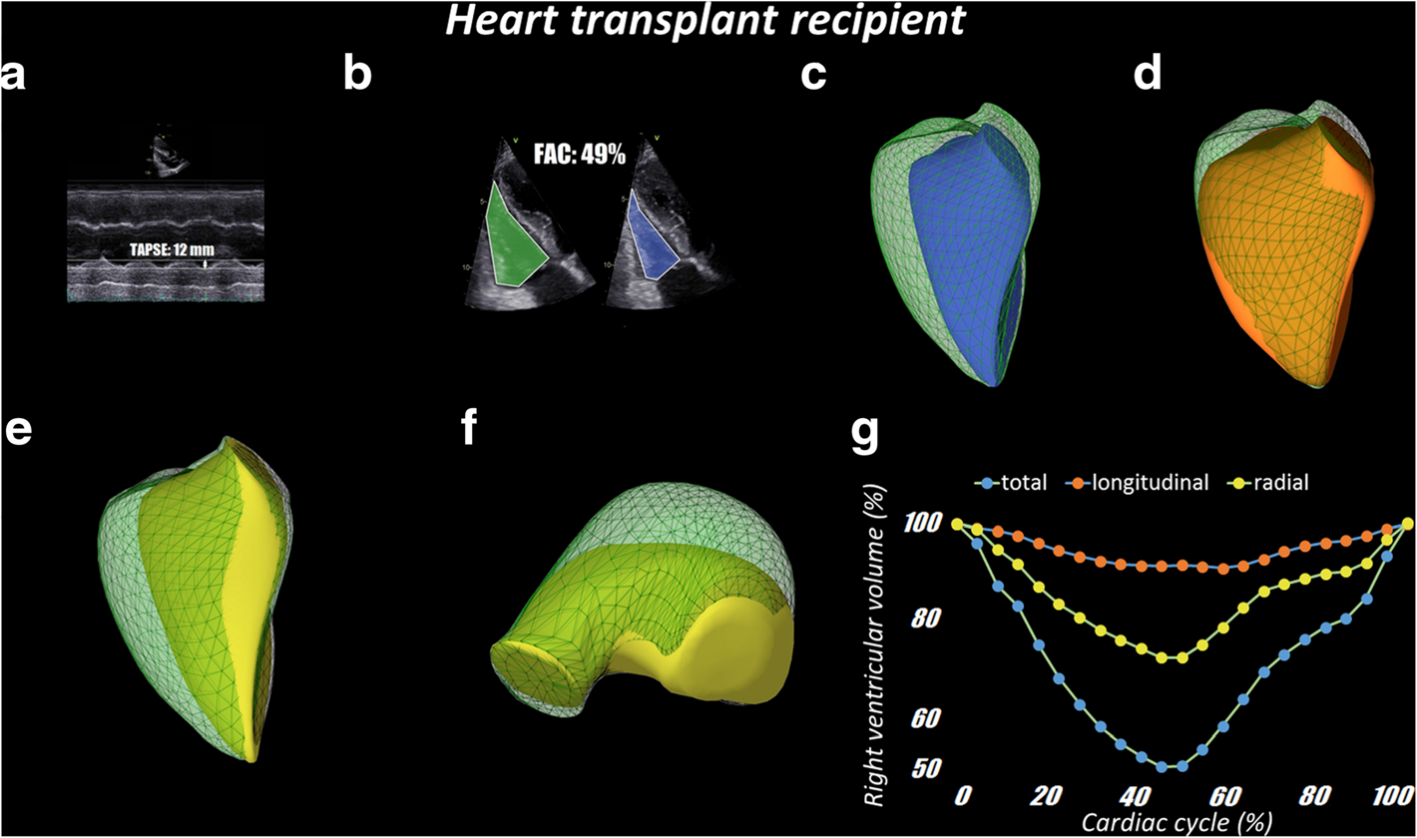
Representative heart transplant recipient. Panel a: TAPSE (12 mm) is reduced indicating moderate RV systolic dysfunction. Panel b: FAC (49%) is preserved indicating normal RV systolic function. Panel c: RV end-diastolic (90 ml) and end-systolic (44 ml) volumes as well as RV ejection fraction (51%) obtained from 3D echocardiography data sets show normal RV systolic function. Panel D: RV longitudinal displacement is markedly reduced. Panel e and f: By removing the longitudinal and anteroposterior components of RV volume change allows the appreciation of the increased extent of radial displacement of RV wall. Panel g: Quantitative analysis of the relative contribution of longitudinal and radial RV wall displacement to global RV volume change confirms the significant reduction of longitudinal displacement and an increase of radial displacement as the main mechanism to preserve RV stroke volume and ejection fraction (patient 144 days after the transplantation)
